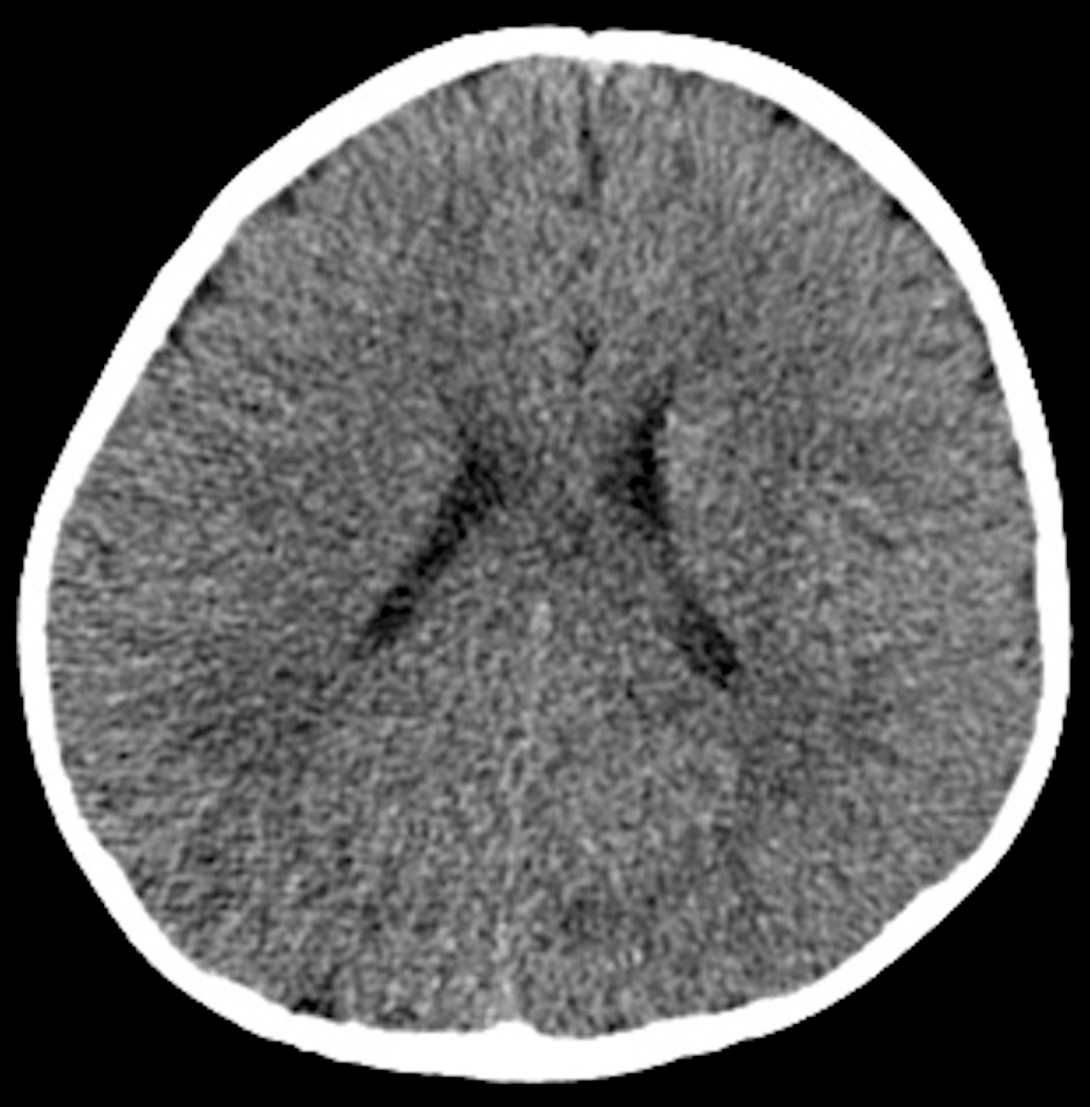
Non-contrast head CT (coronal view) at 10 months of age. No intracranial calcification, hydrocephalus, or other abnormal findings are observed.
